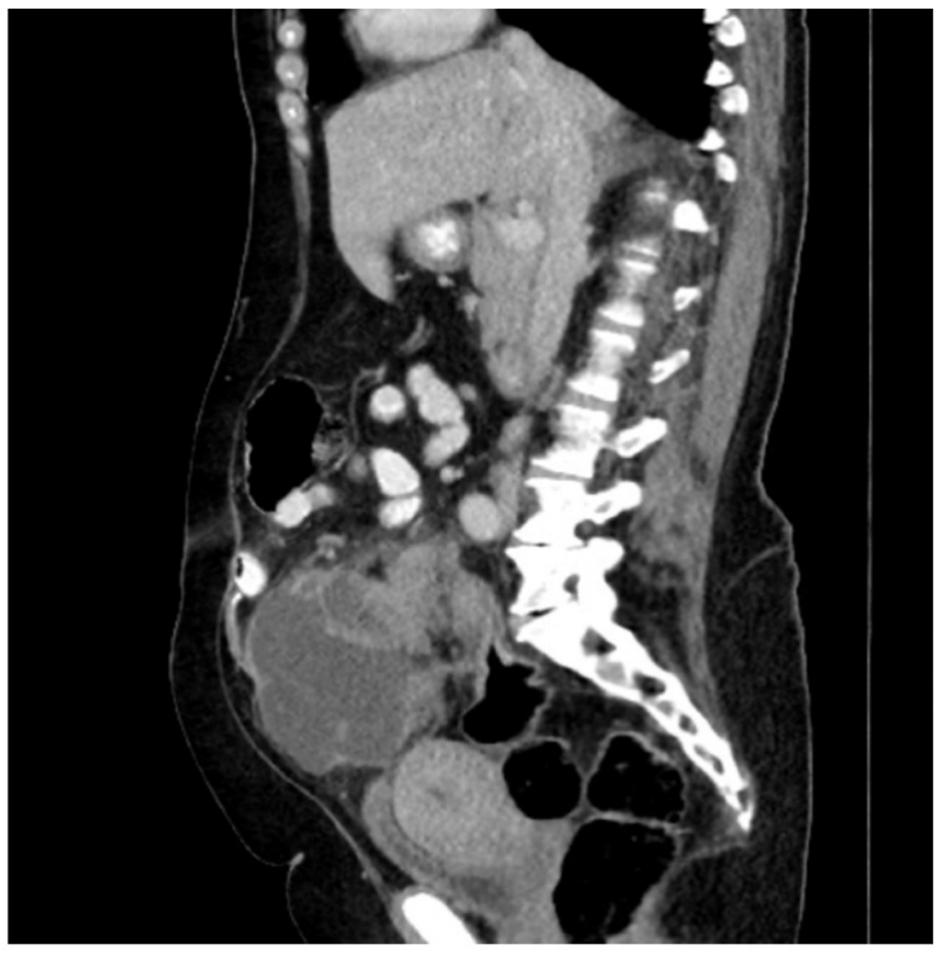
Sagittal CT with mass measuring ~9 cm × 7.5 cm.
